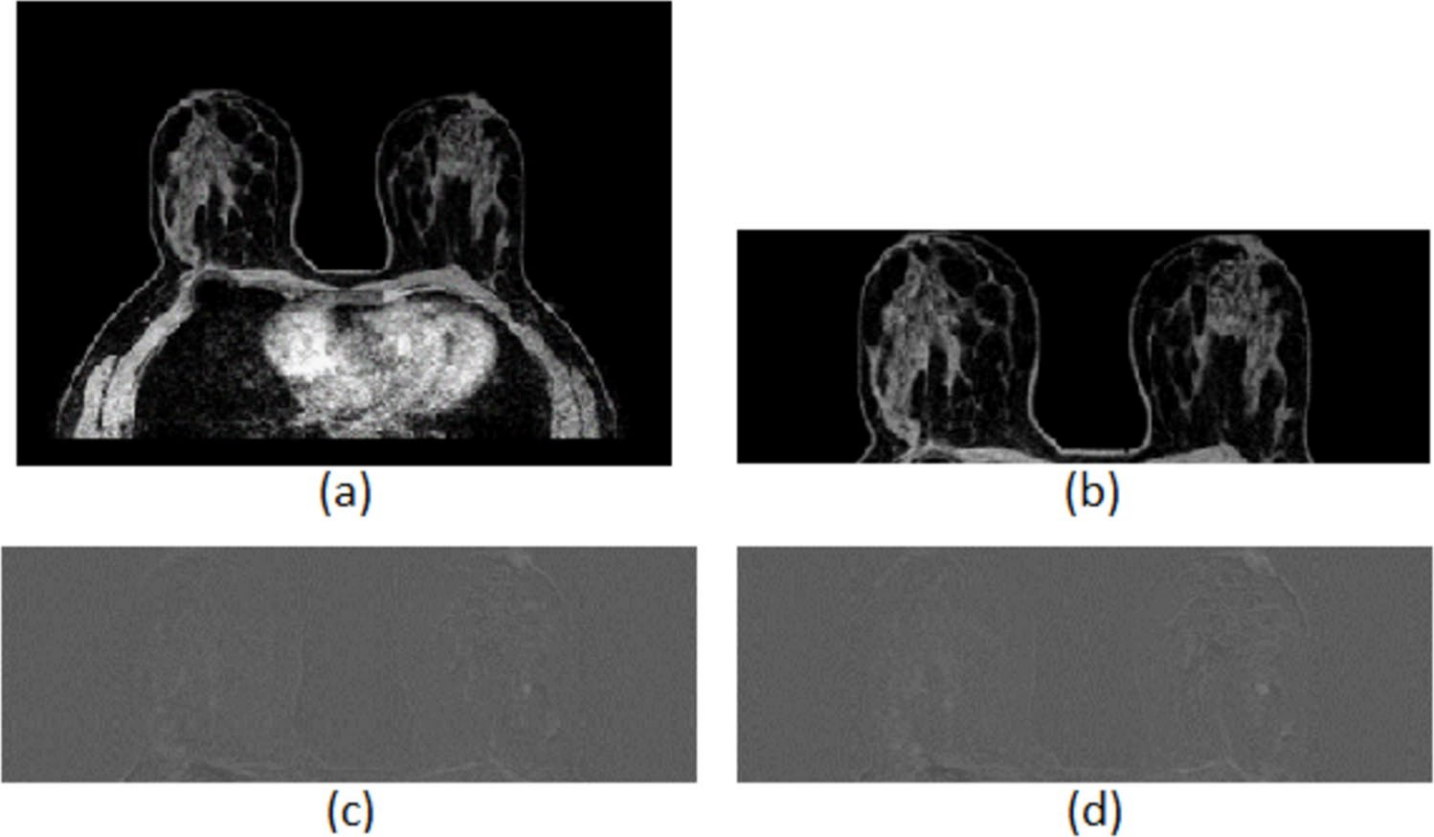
Breast region segmentation: a initial MRI slice, b breast area in the initial MRI slice, c breast area in the second post-contrast subtraction image, and d breast area in the third post-contrast subtraction image
